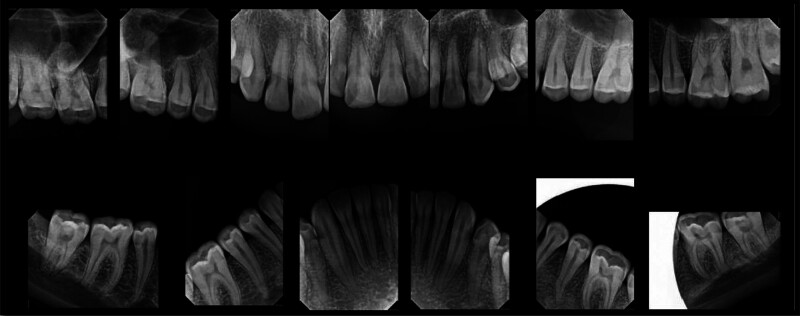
Preoperative Full-mouth x-rays.
